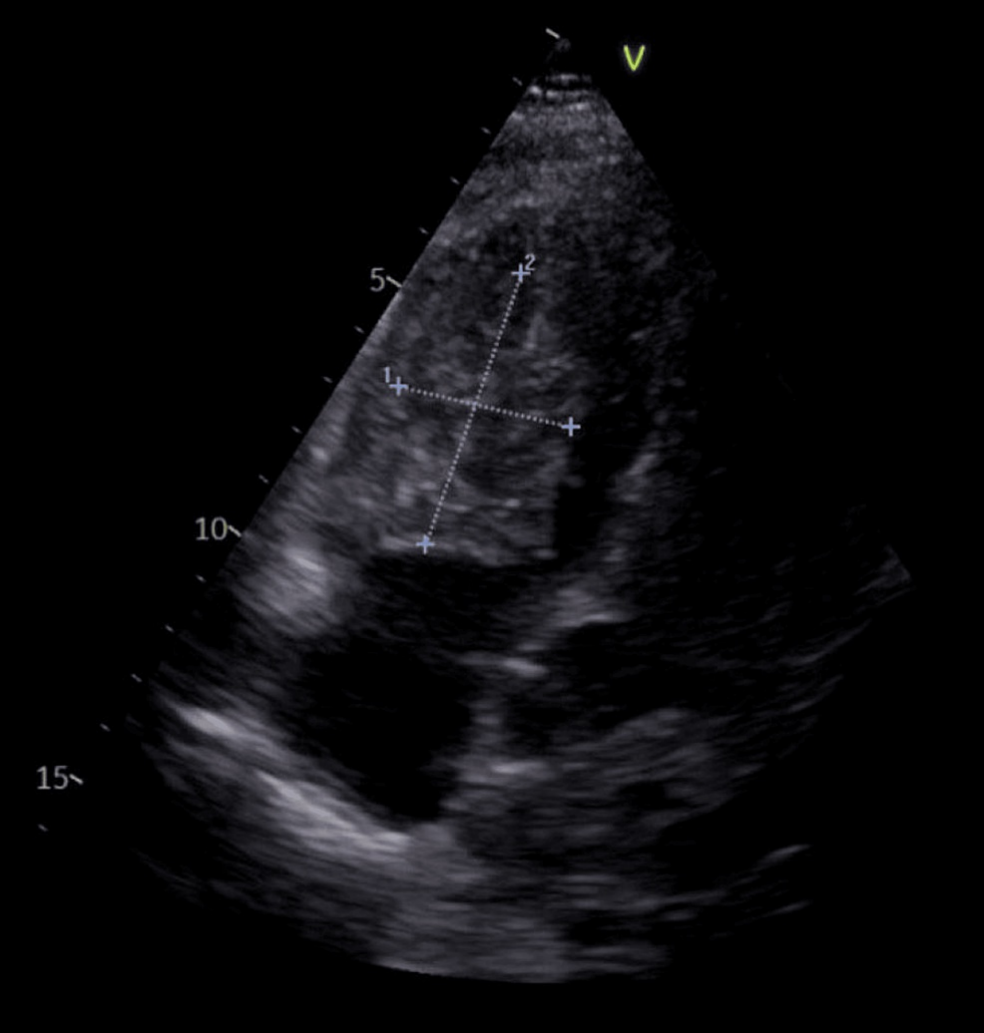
Transthoracic echocardiographic image of the mass.
